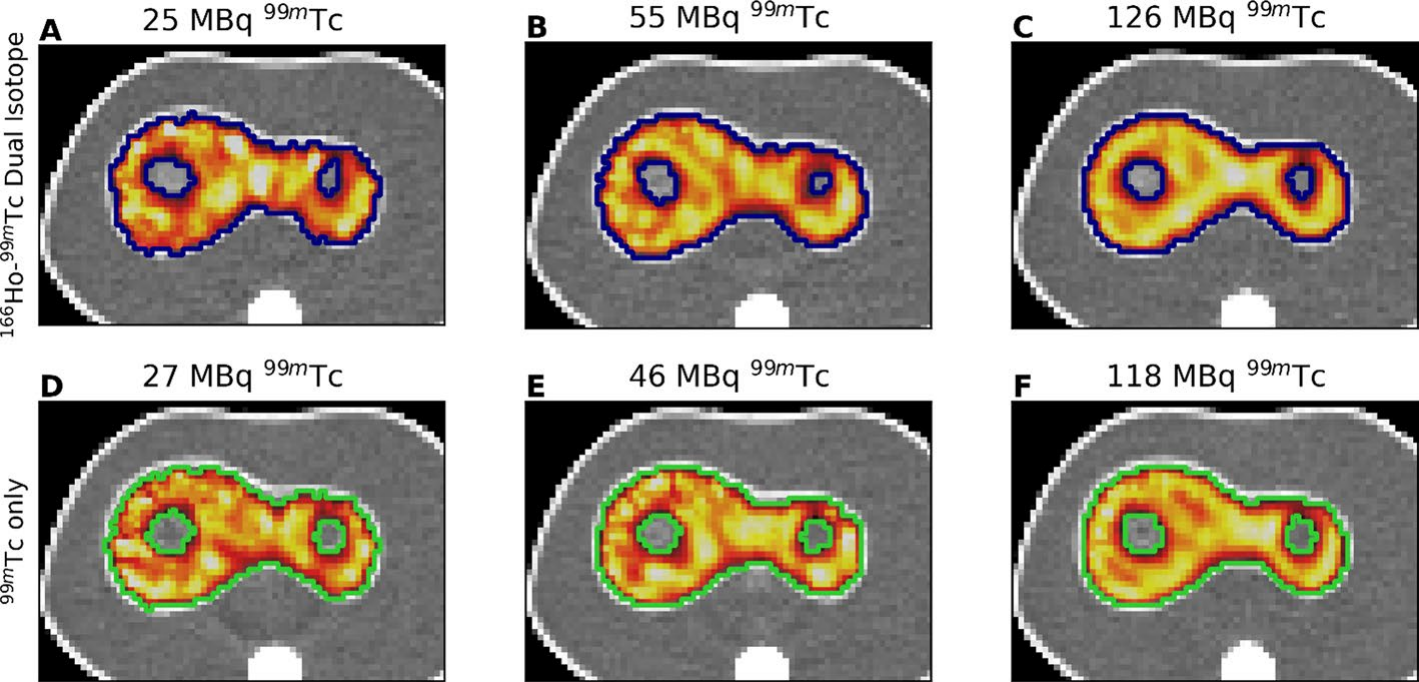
Axial view of ^99m^Tc reconstructions and healthy-liver segmentations. Healthy-liver segmentations of ^99m^Tc SPECT/CTs, acquired in presence of 250 MBq^166^Ho (top panel) and without ^166^Ho (bottom panel). Three ^99m^Tc activity levels are presented to indicate the effect on image quality and segmentation accuracy
